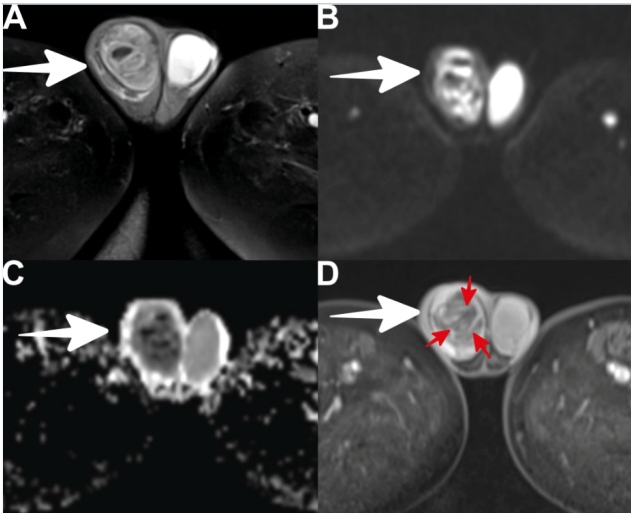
A - Axial T2-weighted images showing an increased size of the right testis, heterogeneous changes in parenchymal signals, and cystic areas (arrow). B and C - Diffusion-weighted images and apparent diffusion coefficient (ADC) maps showing diffusion restriction of the right testis (arrow). D - Axial contrast-enhanced images showing the loss of contrast enhancement (arrow) and areas of septal enhancement (red arrows) in the right testis.
